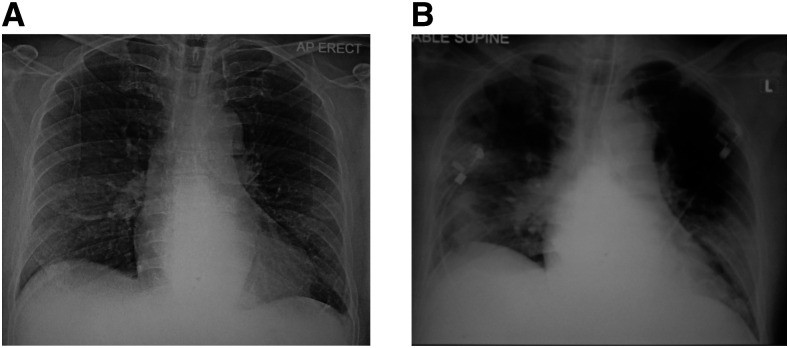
Chest radiographs. (A) Day 3 of hospitalization which was normal and (B) day 5 which showed bilateral consolidations.
